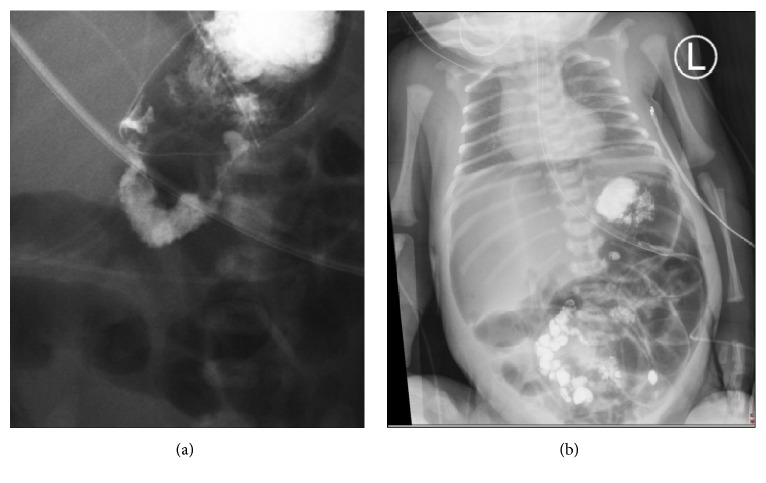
Radiographic images from day 1 and day 2 postnatally. (a) Contrast study performed on day 1 of life excluding malrotation and (b) large pneumoperitoneum evident on abdominal X-ray on day 2 of life.
